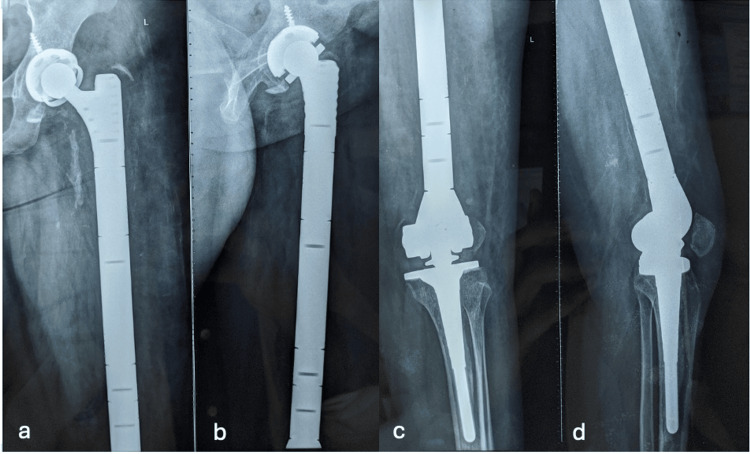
Four years follow up radiographs showing the constrained uncemented acetabular component and the total femur prosthesis a. X-ray (anteroposterior view) of hip showing constrained uncemented acetabular component; b. X-ray (lateral view) of hip showing constrained uncemented acetabular component; c. X-ray (anteroposterior view) of knee showing linked prosthesis component; d. X-ray (lateral view) of knee showing linked prosthesis component.
